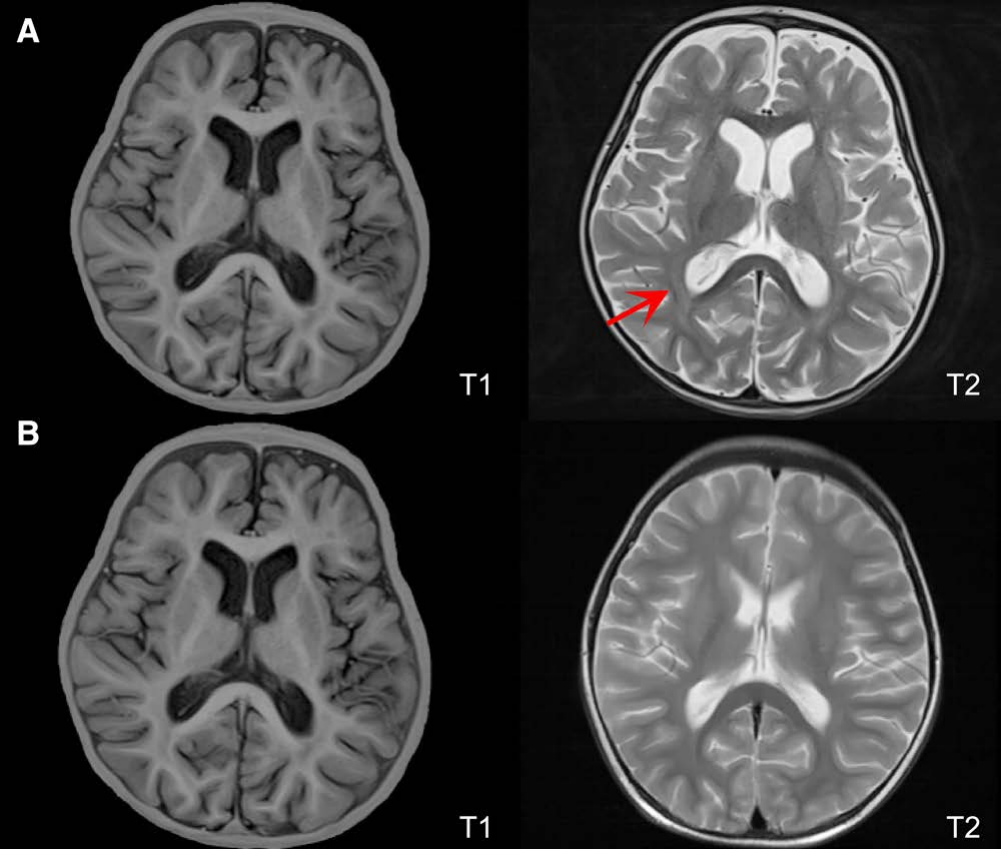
Cranial MRI result of the patient. A: Cranial MRI (on admission): Slightly wide bilateral lateral ventricles, slightly wide and deepened sulcal fissures, slightly wide bilateral temporal pole extracellular space, abnormal signals in the white matter area next to the lateral ventricles; B: Reexamination of cranial MRI (20 d after admission): Slightly wide sulcus in part of bilateral cerebral hemispheres and disappearance of abnormal signal in the paraventricular white matter area. MRI = magnetic resonance imaging.
